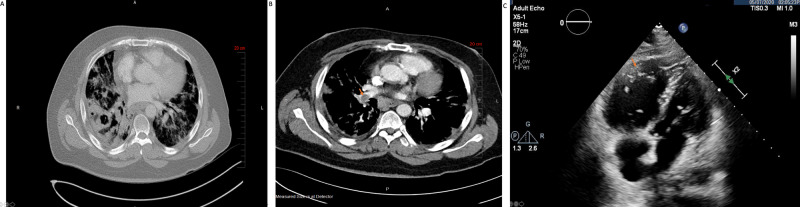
Pulmonary Thromboembolism in a Patient with Severe COVID-19 A large pulmonary embolism in the right pulmonary artery is seen in computerized tomography angiography which extends to the upper, lower, and middle lobar arteries and multiple segmental branches. In addition, there is a right lower lobe cavity lesion (A). There is diffuse bilateral ground glass-like interstitial airspace disease in the lungs (B). Transthoracic echocardiogram showing severely dilated right ventricle and severely decreased right ventricular systolic dysfunction (C).
